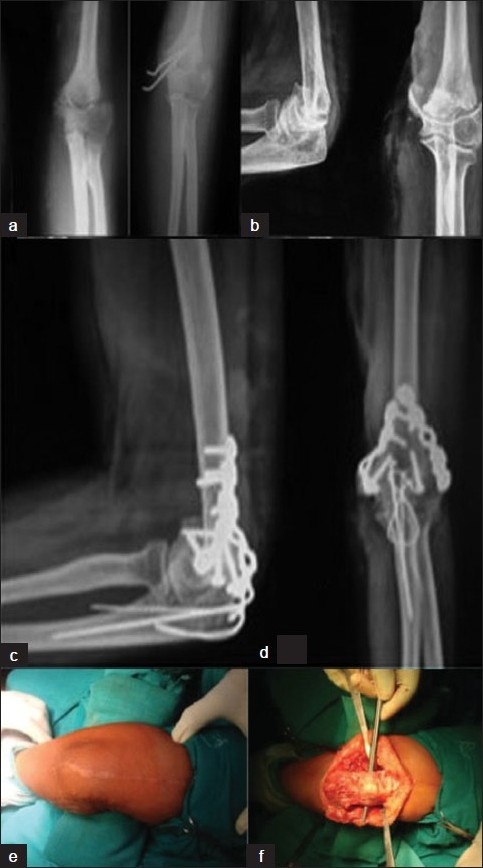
Plain X-ray anteroposterior view (a) shows intraarticular fracture of distal humerus that was treated by K-wire fixation (b); developed nonunion (c); nonunion stabilized by bicolumnar fixation with reconstruction plates and extra-articular olecrano-osteotomy stabilized by tension band wiring (d). Removal of implant was planned by paratricipital incision (e); the first picture shows the postoperative scar; the second picture (f) shows a paratricipital incision for removal of implant.
